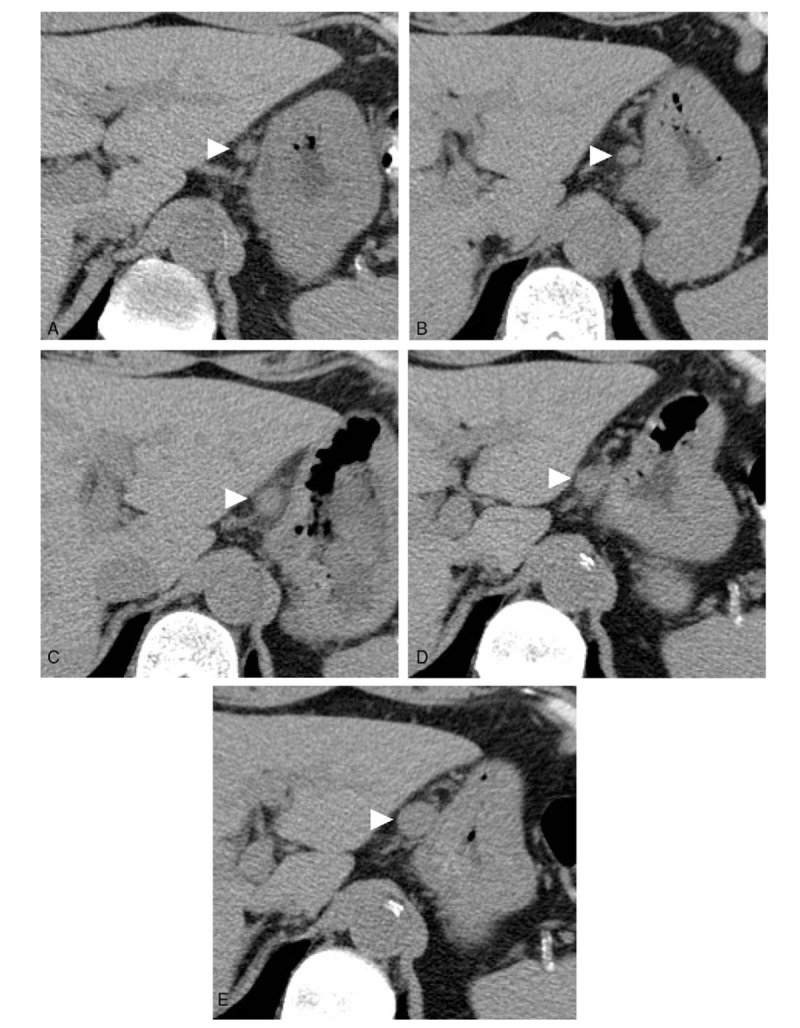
Gradual enlargement of the mass as shown by annual plain CT. The mass of interest (arrowhead) measured 10 × 8 mm in diameter in June 2013 (A), 10 × 9 mm in July 2014 (B), 16 × 10 mm in July 2015 (C), 16 × 11 mm in June 2016 (D), and 18 × 14 mm in June 2017 (E). These images show a gradual and clear increase in the tumor size. CT = computed tomography.
